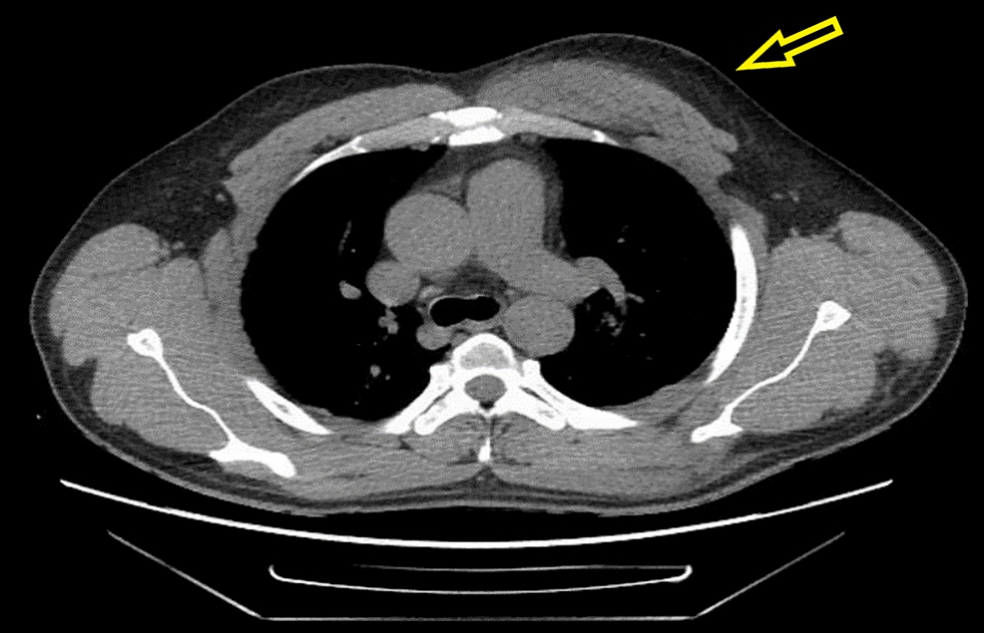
CT scan of the chest showing diffuse edema (yellow arrows) within the left chest wall compartment.
